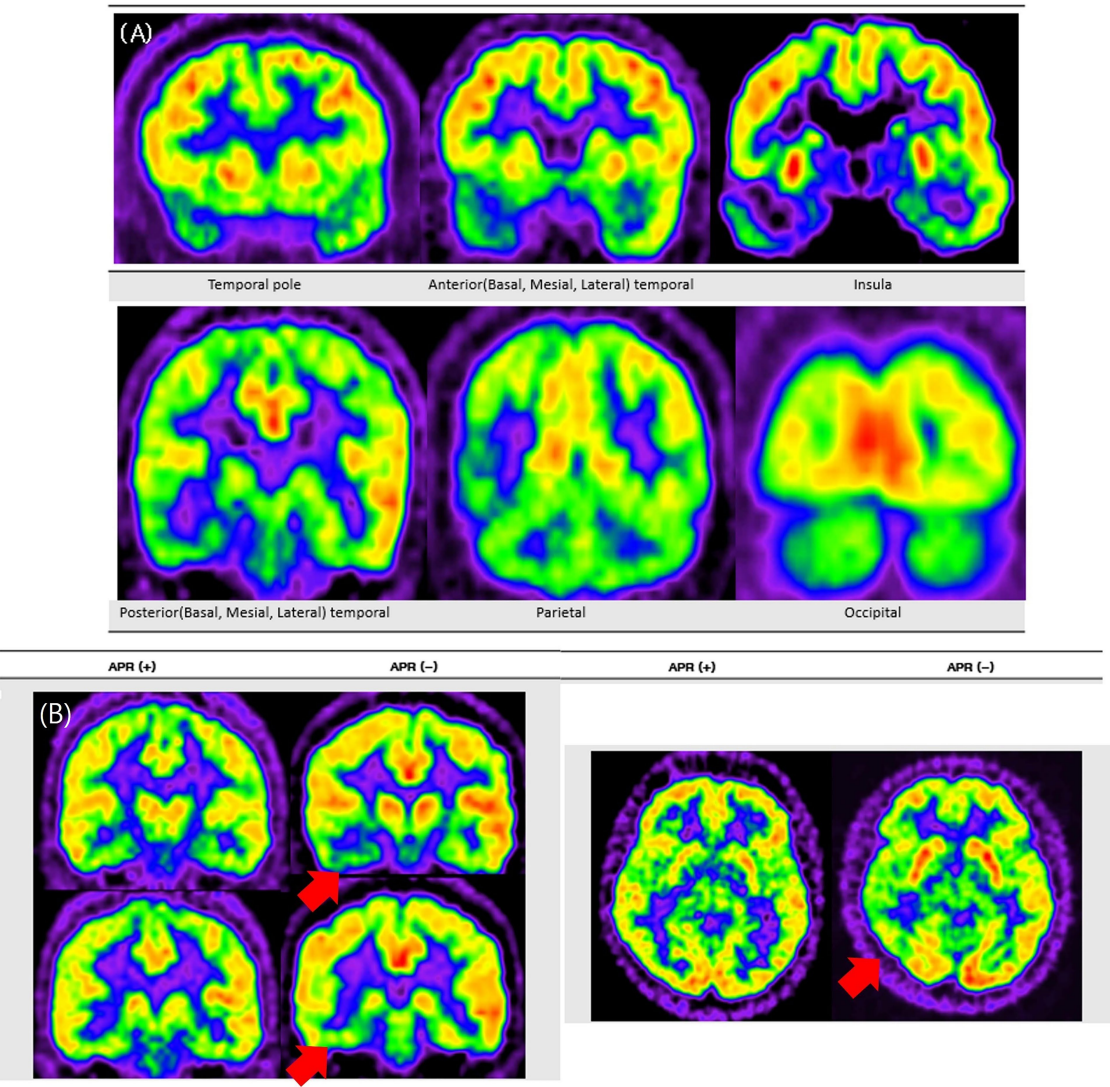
Representative FDG-PET images contrasting APR− and APR+ patients. This figure presents FDG-PET images comparing patients with and without preserved ictal responsiveness (APR+ and APR−). Panel A shows a typical pattern of extensive hypometabolism in a representative patient with right mesial temporal lobe epilepsy, involving the temporal pole and extending to temporal, parietal, insular, and occipital regions. Panel B shows two patient examples, where red arrows indicate more pronounced ipsilateral posterior temporal hypometabolism in the APR− patient compared to the APR+ patient.
